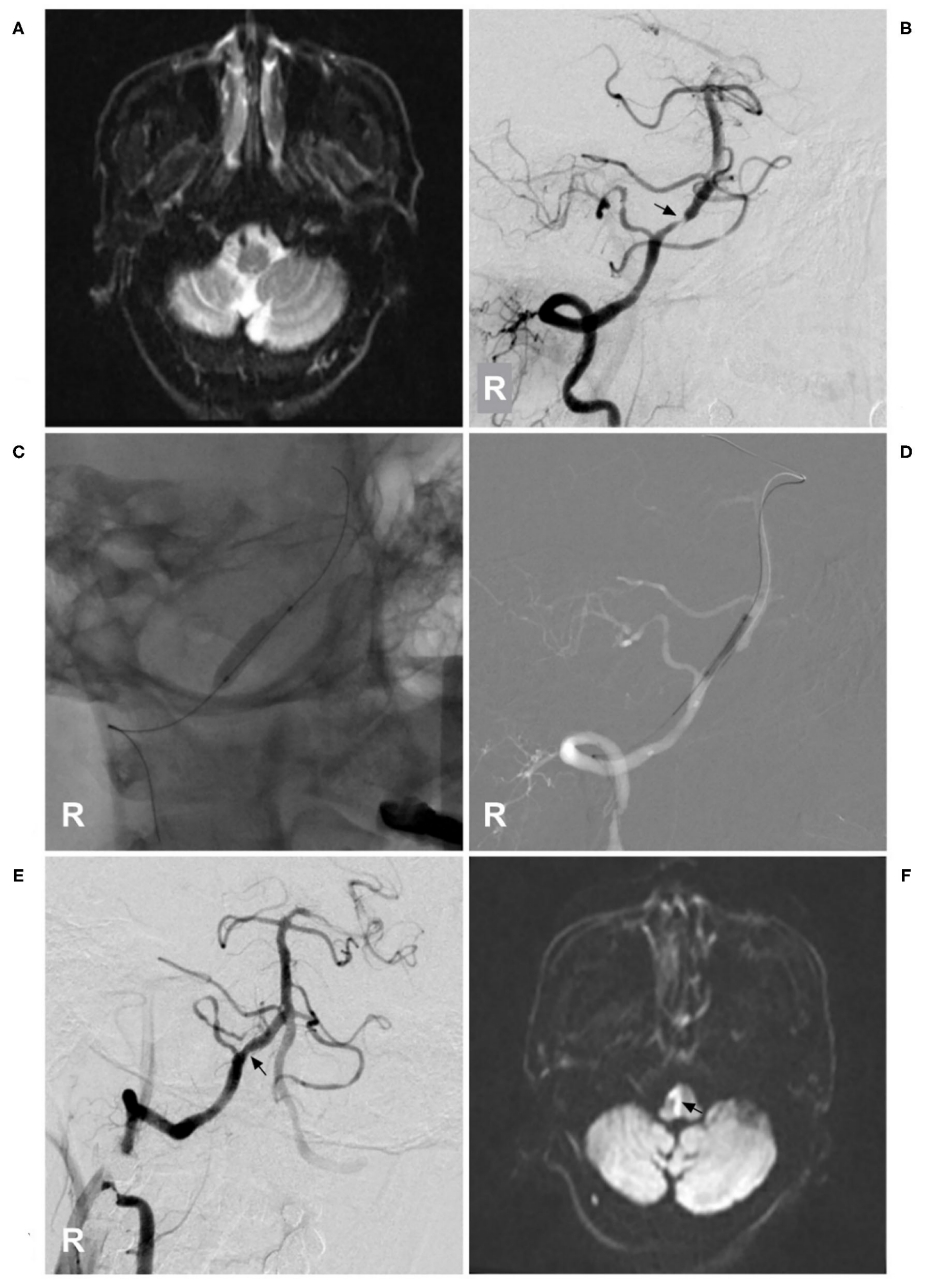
Medulla oblongata infarction from ASA ischemia after stent angioplasty. (A) MRI showed a normal medulla oblongata. (B) A right VA angiogram showed stenosis (arrow) of the VA beyond the posterior inferior cerebellar artery. (C) Balloon angioplasty was performed. (D) A balloon-expandable stent was deployed. (E) After stent angioplasty, the stenosis was relieved (arrow). (F) Postoperative MRI showed acute medulla oblongata infarction (arrow), indicating ASA ischemia and resulting in locked-in syndrome. ASA, anterior spinal artery; MRI, magnetic resonance imaging; R, right; VA, vertebral artery.
